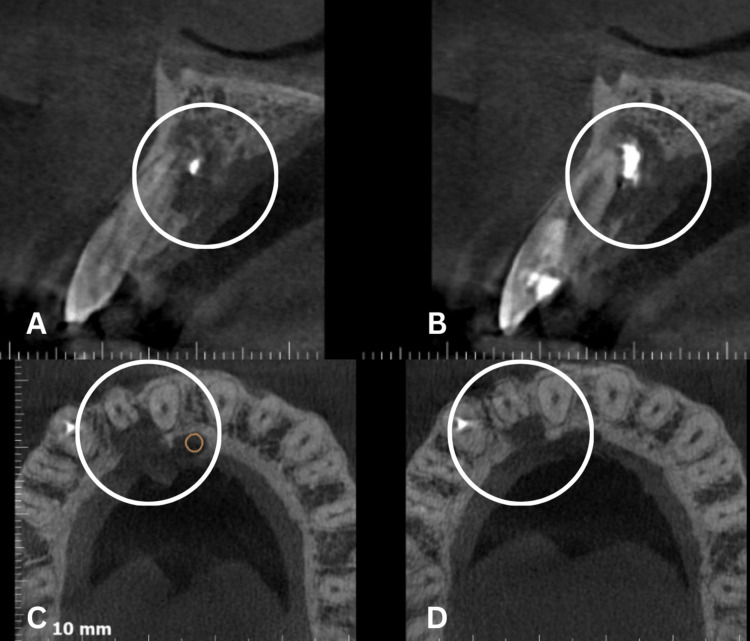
Pre-operative CBCT image A: Pre-operative cross-sectional CBCT scan demonstrating the extent of the lesion. B: Pre-operative cross-sectional CBCT scan showing another view of the lesion. C: Pre-operative axial CBCT scan highlighting the lesion. D: Pre-operative axial CBCT scan providing another view of the lesion.
